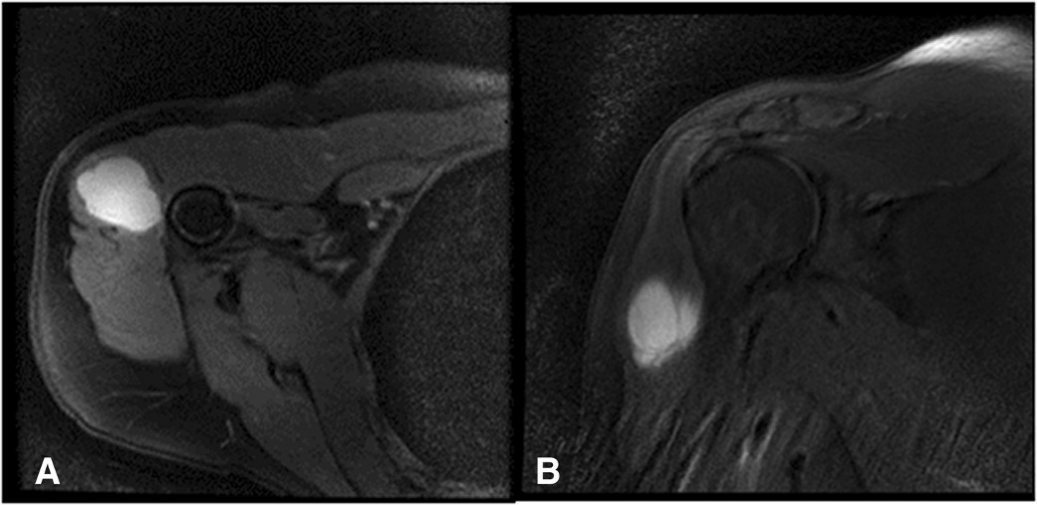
Appearance of the mass on T2-weighted magnetic resonance imaging sections. A) In axial section; B) in coronal section.
